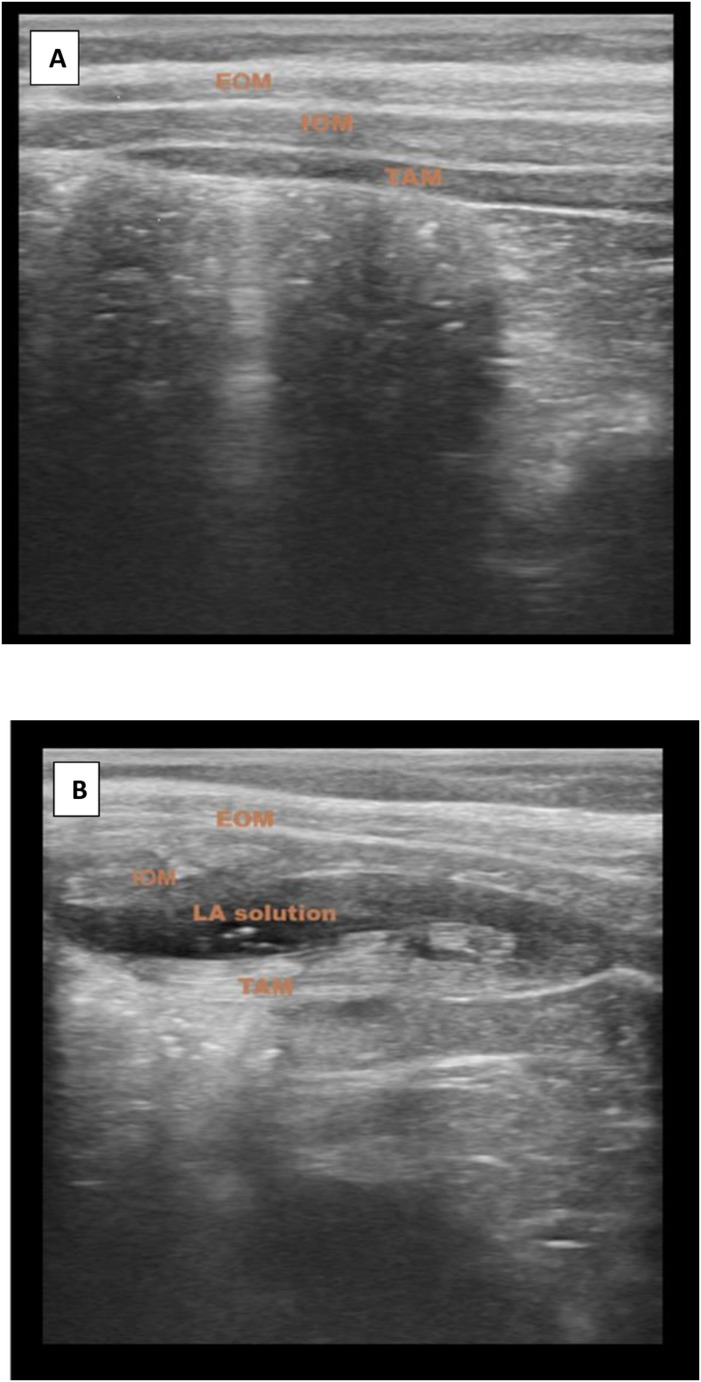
A Tap Block USG image (before injection). B Tap Block USG image (distribution of local anesthetic after injection). Ultrasonography image showing the muscle layers of the abdominal wall. EOM: External oblique muscle, IOM: Internal oblique muscle, TAM: Transversus abdominis muscle, LA: Local anesthetics
